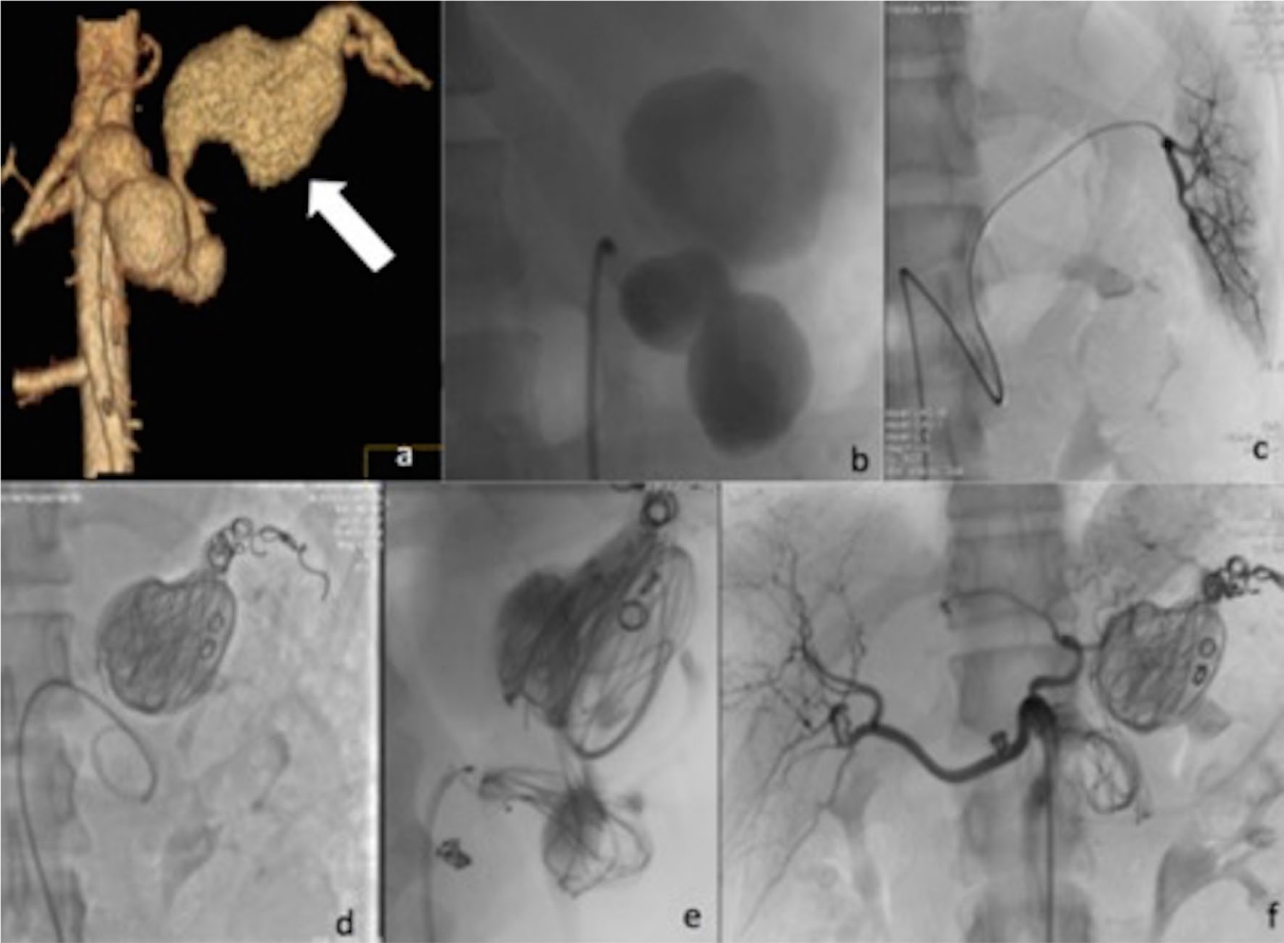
(a) Volume rendering contrast enhanced CT confirming the presence of multilobed fusiform splenic artery GVAA measuring 88 × 140 mm (arrow). (b) Angiogram confirmed the CT findings. (c) Selective catheterization of the distal splenic artery of “back door” of the aneurysm. (d,e) Endovascular exclusion was obtained by transcatheter “sandwich” embolization with Tornado coils (Cook Medical, Bloomington, Indiana, USA) and Jackson coils (Cook Medical, Bloomington, Indiana, USA) of the distal and proximal tract of the main artery and packing of the sac. Furthermore, inflow was totally stopped using a 8 mm detachable vascular plug (Amplatzer Plug, AGA, Plymouth, USA) in the proximal neck of the aneurysm. (f) Angiogram from the coeliac axis confirming satisfactory exclusion of the GAA.
